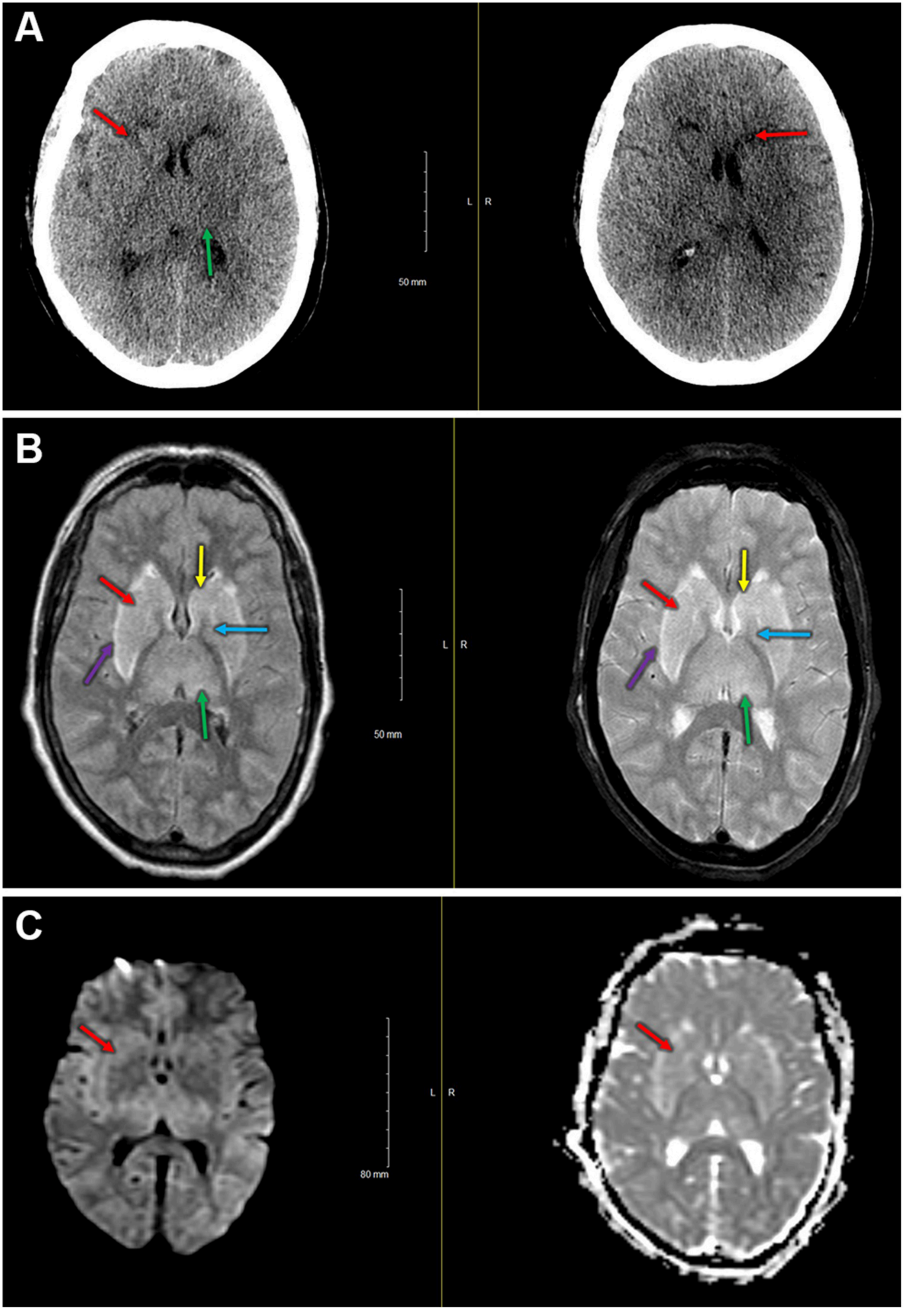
(A) Immediate postoperative non-contrast head CT shows bilateral symmetric hypoattenuation adjacent to the basal ganglia (red arrows). Less pronounced findings throughout the thalamus (green arrow). (B) Left—fluid attenuation inversion recovery imaging shows bilateral hyperintense signals in the basal ganglia (caudate [yellow] and putamen [red], globus pallidus [blue]) and in the thalami [green]). Note the hyperintense signal at the external capsule [purple], just lateral to the putamina. Right—T2 fat suppressed imaging demonstrating hyperintense signal in the basal ganglia (caudate [yellow] and putamen [red], globus pallidus [blue]) and in the thalami [green]). Hyperintense signal also present in the external capsules [purple]. (C) Diffusion-weighted imaging (left) and apparent diffusion coefficient (right) demonstrate slightly elevated signal on diffusion-weighted imaging with small areas of hypointense signal on the apparent diffusion coefficient in the basal ganglia (i.e., right anterior putamen, red arrow).
